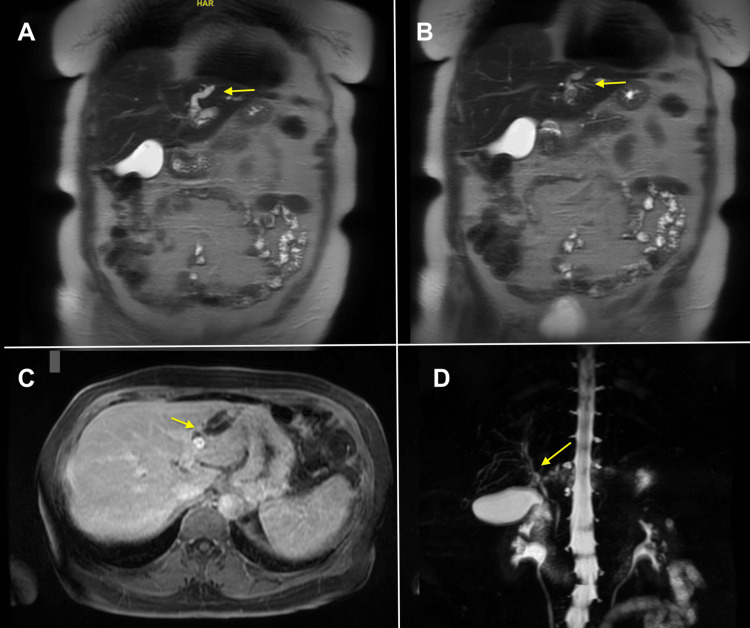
MRCP images (A, B): T2-weighted images with dilated CBD (yellow arrows). (C) Axial volumetric interpolated breath-hold examination/liver imaging with volume acceleration (VIBE/LAVA) showing intrahepatic biliary dilation (yellow arrow). (D) Coronal view demonstrating intrahepatic biliary dilation (yellow arrow). MRCP: magnetic resonance cholangiopancreatography, CBD: common bile duct.
